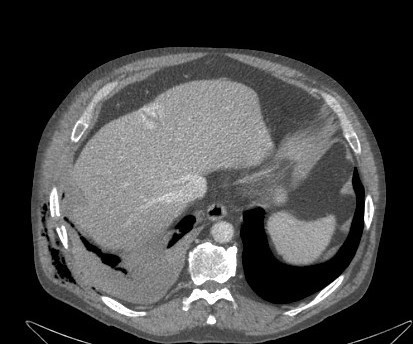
CT scan on admission. Fracture of the ninth right rib with hematothorax and emphysema.
